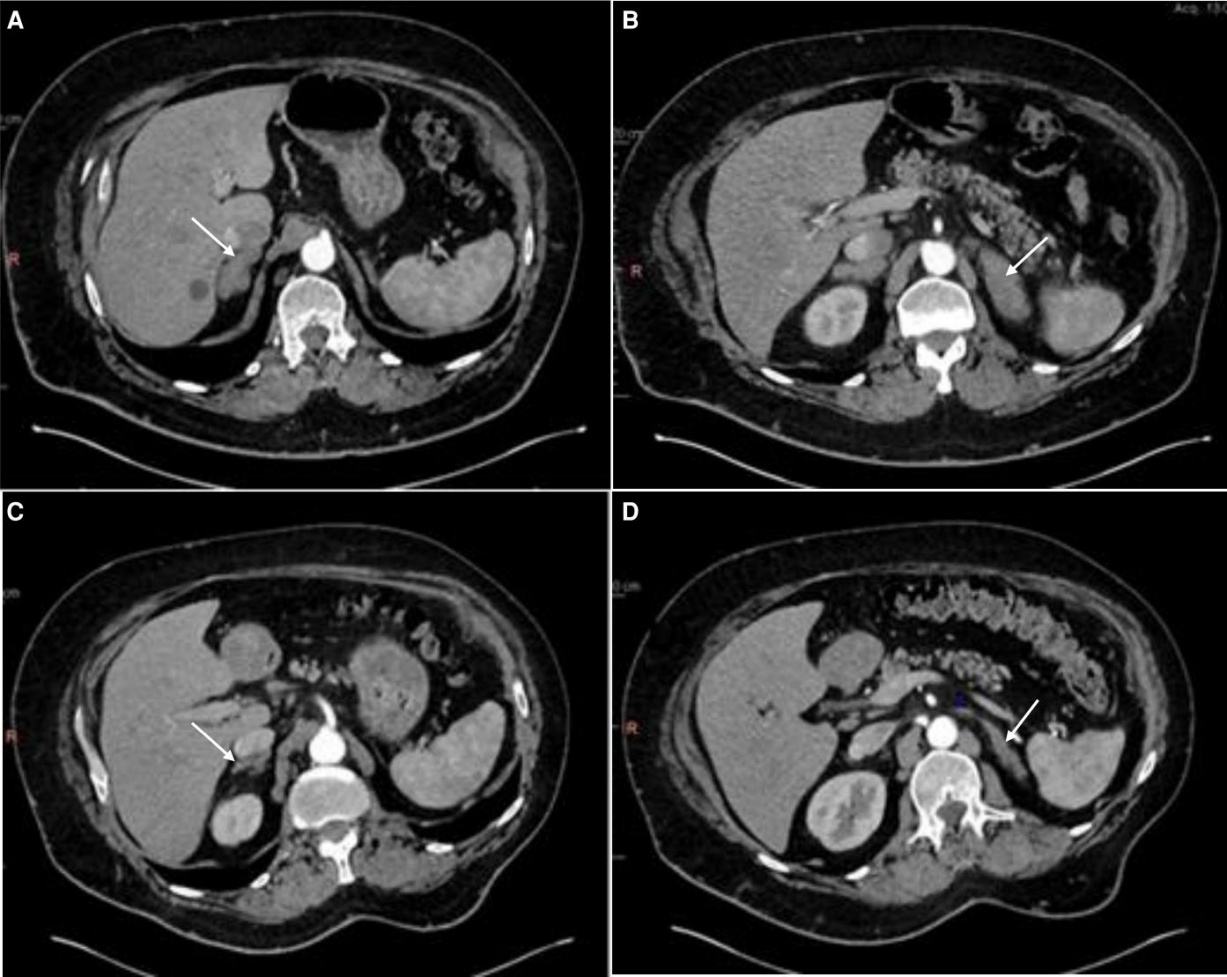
Contrast CT scan shows adrenal thickening of the right (A) and left (B) adrenal consistent with immune checkpoint inhibitors-associated adrenalitis. (C) and (D) show adrenal thickening resolution. White arrows highlight both adrenal glands. CT scans were performed on a 128-slice machine with an arterial time acquisition [start of acquisition 10 seconds from the optimal enhancement of the region of interest (at 100 Hounsfield Units) at the upper abdominal level and a venous phase thoraco-abdominopelvic acquisition (venous phase: 30 seconds from the end of arterial acquisition)]. Abbreviation: CT, computed tomography.
